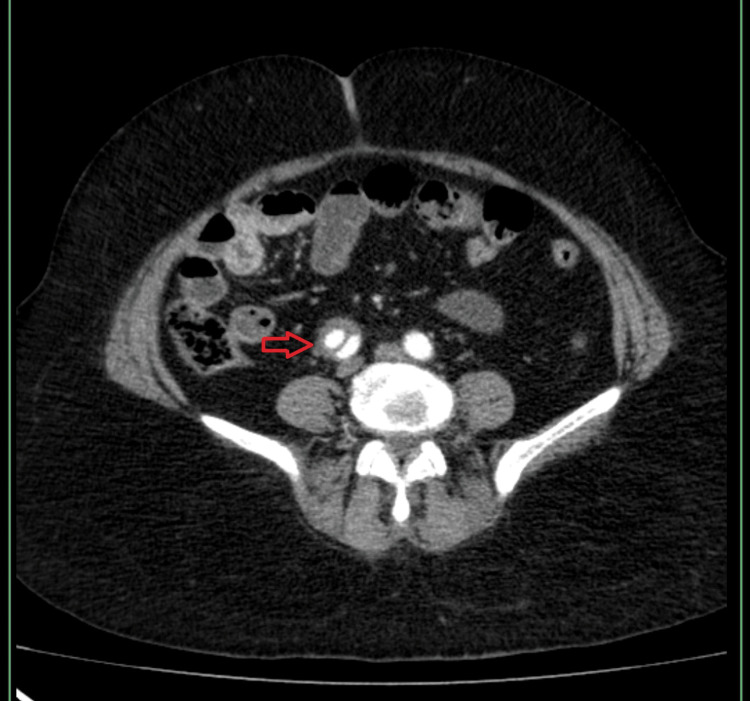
Axial contrast-enhanced CTA demonstrating distal extension of the infrarenal aortic dissection The image shows a persistent intimal flap (red arrow) within the distal infrarenal aorta, maintaining a double-lumen appearance and confirming distal propagation of the dissection toward the iliac arteries. CTA, CT angiography
